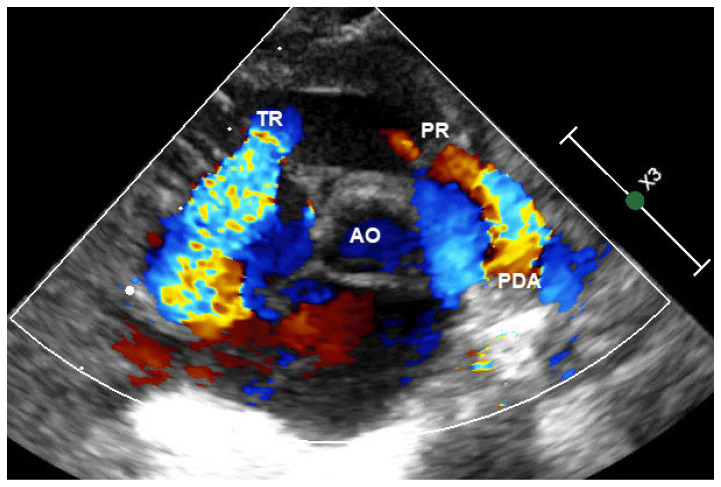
Echocardiographic findings in a neonate with functional pulmonary atresia at admission. Color Doppler imaging demonstrates a thick patent ductus arteriosus (PDA) with turbulent pulmonary arterial flow, while no antegrade blood flow across the pulmonary valve (PV) is observed. Abbreviations: PDA, patent ductus arteriosus; PV, pulmonary valve.
